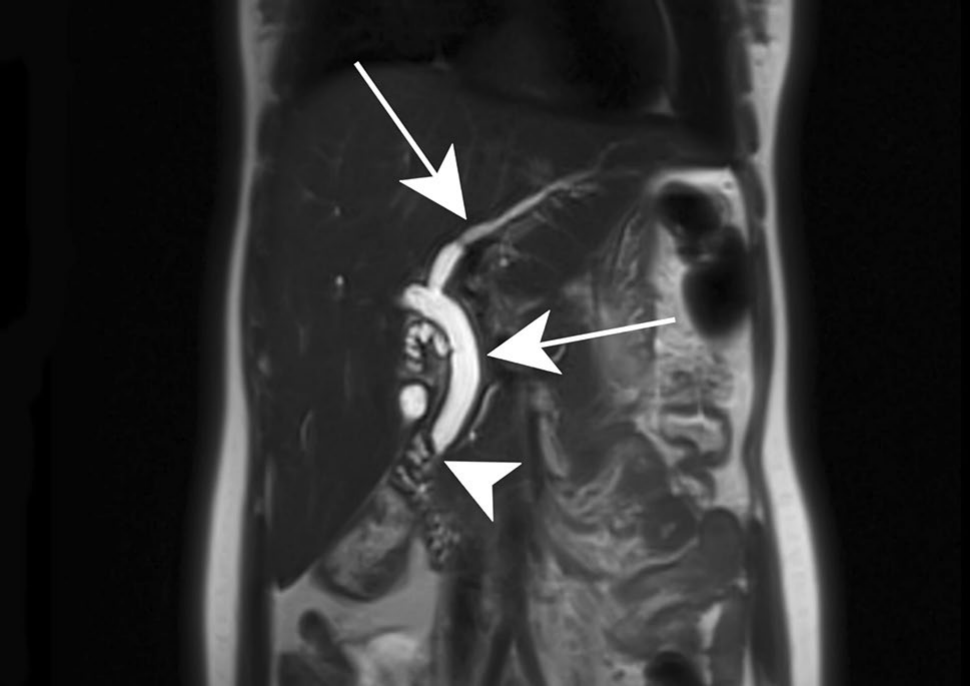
HIV-related cholangiopathy in a 16-year-old female patient. Coronal T2-weighted image demonstrates intrahepatic and extrahepatic bile duct dilatation (white arrows) due to papillary stenosis (white arrowhead), a common finding in this disease
